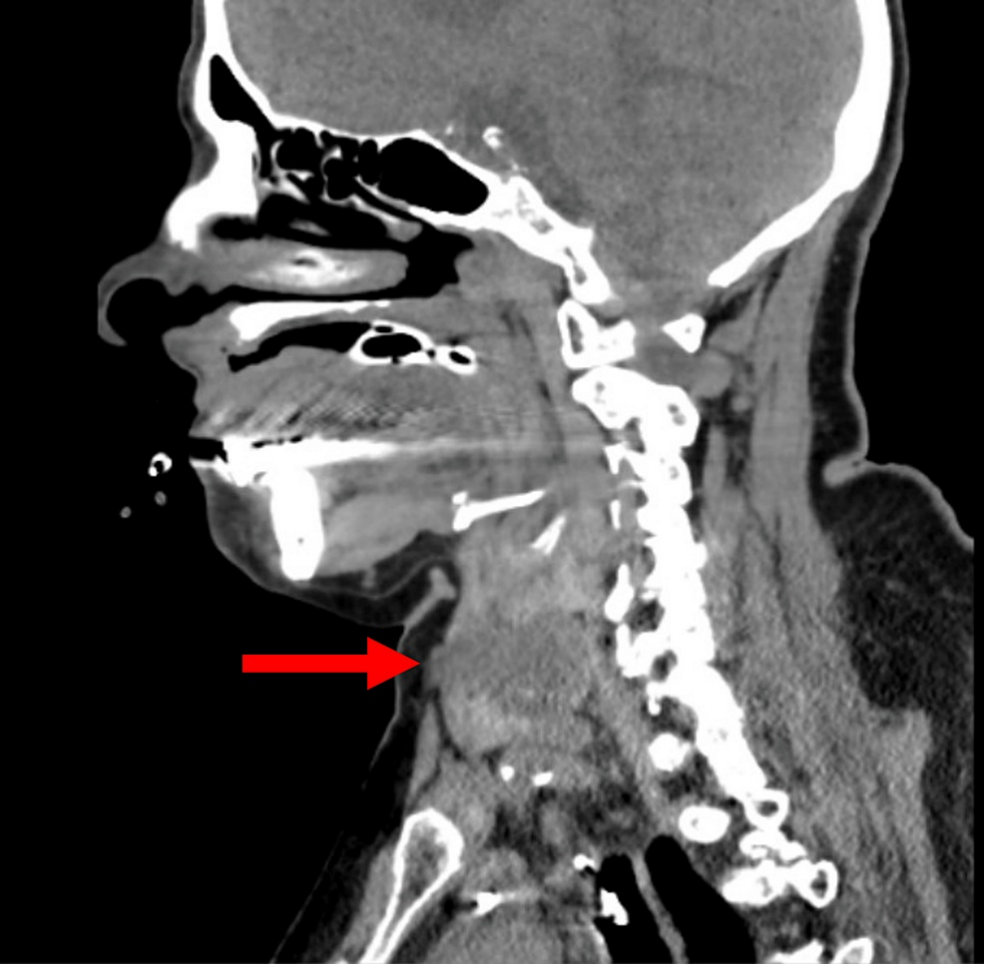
Computed tomography neck and soft tissue without contrast: notable for a heterogeneous thyroid with a focal nodule in the right thyroid lobe measuring up to 3 centimeters (red arrow)
